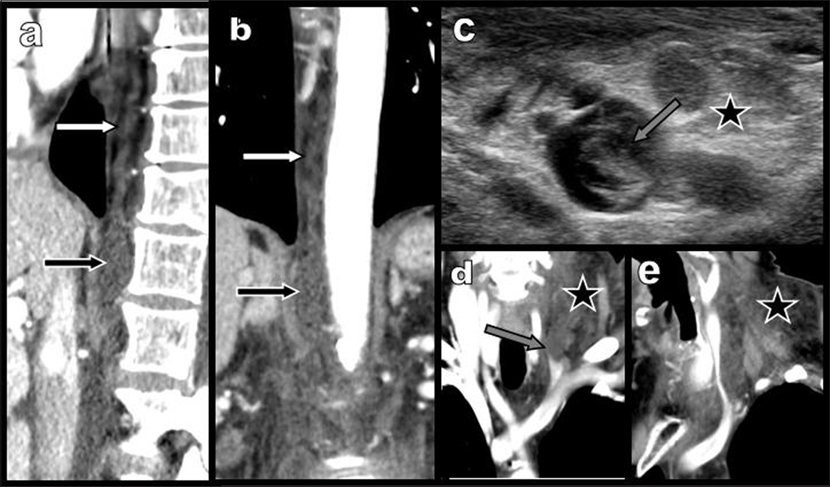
Case 2: Sagital (a) and coronal (b) reconstructions at the level of the retrocrural spaces of the diaphragm show distension of the cisterna chyla (black arrow) and a large tortuous thoracic canal (white arrow). Transverse ultrasound view (c) and the corresponding contrast-enhanced coronal CT reconstructions (d) and (e) at the level of the swollen supra-clavicular fossa show massive oedema (black star) and deformation and laminating of the left jugular vein by a tortuous hypoechoic serpiginous structure, probably representing the jugular anastomosis of an ectasic congestive thoracic duct (grey arrow).
